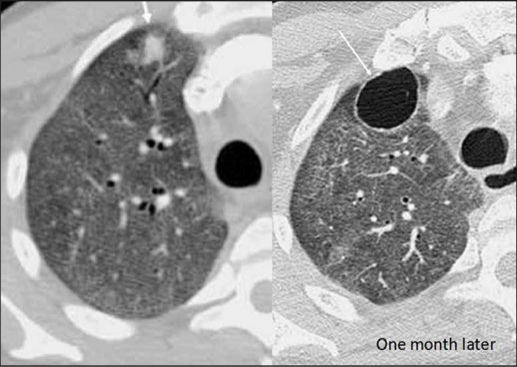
Pneumocystis jirovecii *infection.* A 40-year-old male was admitted with acute dyspnea. An initial CT was done for suspected PE. It showed extensive ground glass opacities in both lungs and a small consolidation in the right upper lobe. The patient was subsequently diagnosed with acquired immunodeficiency. One month later (HRCT) the ground glass is less extensive, and the nodule has developed into a thin-walled cyst.
